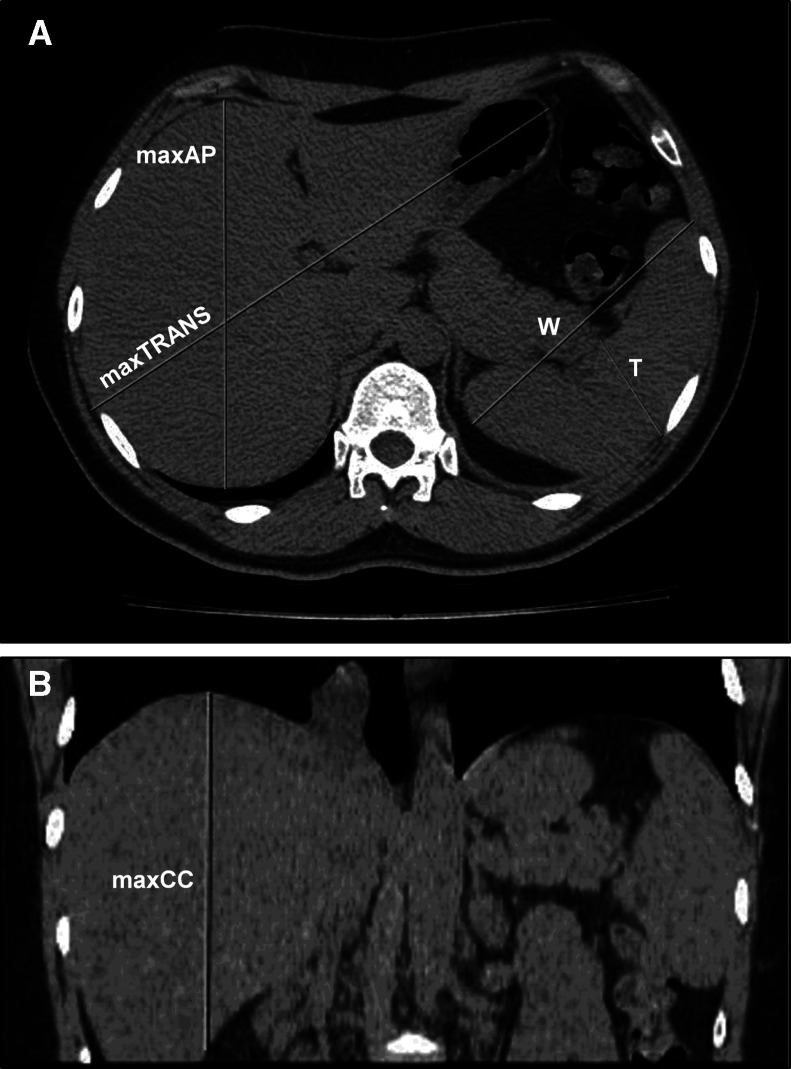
Detection of hepatomegaly and splenomegaly using computed tomography (CT) scan. CT abdomen axial (A) and coronal (B) sections showing the maximum anteroposterior (maxAP = 15.84 cm), transverse (maxTRANS = 22.27 cm), and craniocaudal distances (maxCC = 13.41 cm) of the liver and the maximum splenic width (W = 12.15 cm) and thickness (T = 4.56 cm). The splenic craniocaudal distance (CC = 10 cm) was evaluated using the cranial-most and caudal-most ends of the spleen on the axial sections. Volumes of 1,819.4 cm^3^ and 554.04 cm^3^ for the liver and spleen, respectively, were calculated. This figure appears in color at www.ajtmh.org.
